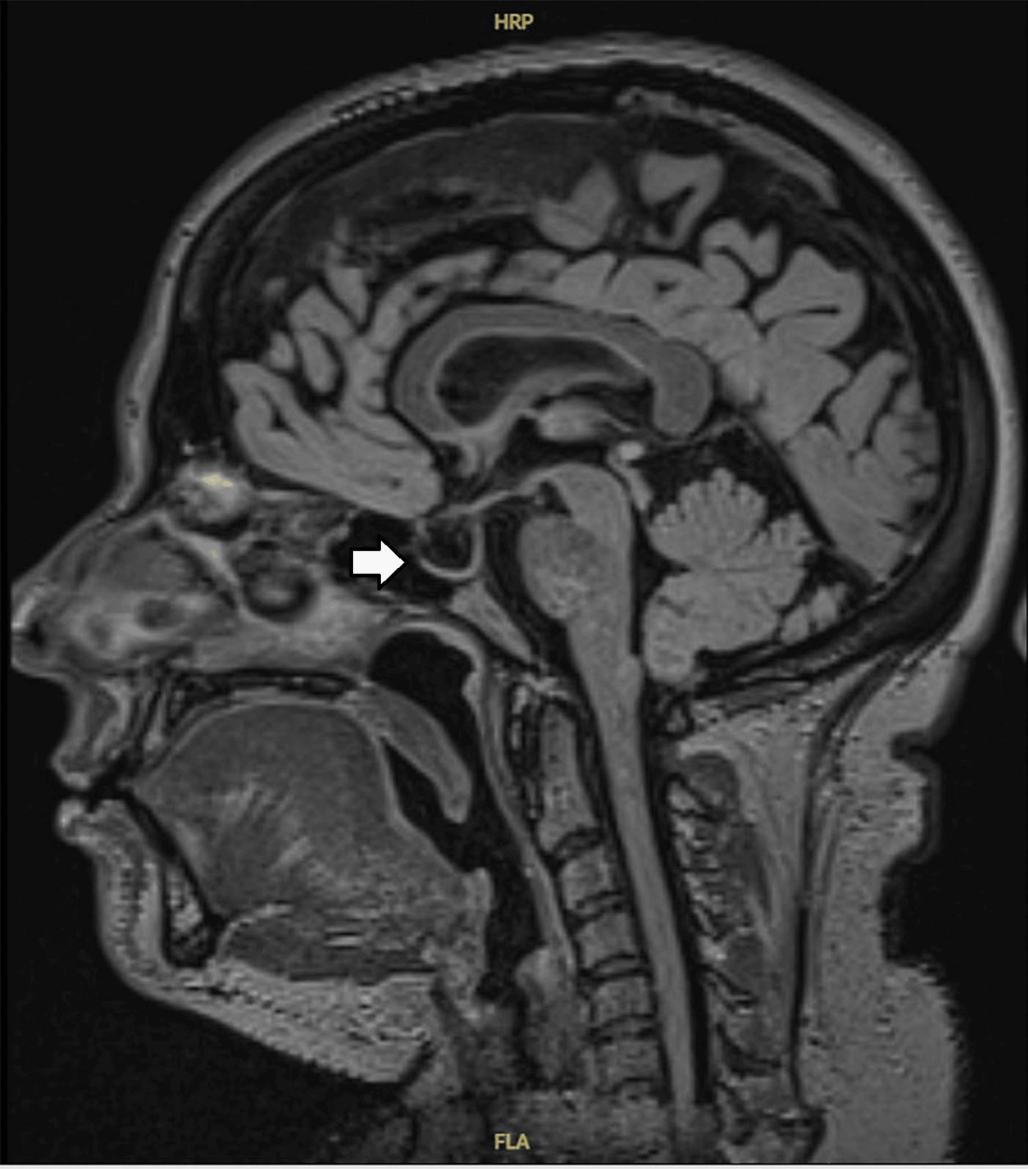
Sagittal T1-weighted MRI brain demonstrating features of empty sella, which appears partially filled with cerebrospinal fluid, resulting in a flattened pituitary gland against the floor of the sella-an imaging feature suggestive of idiopathic intracranial hypertension. MRI: magnetic resonance imaging
